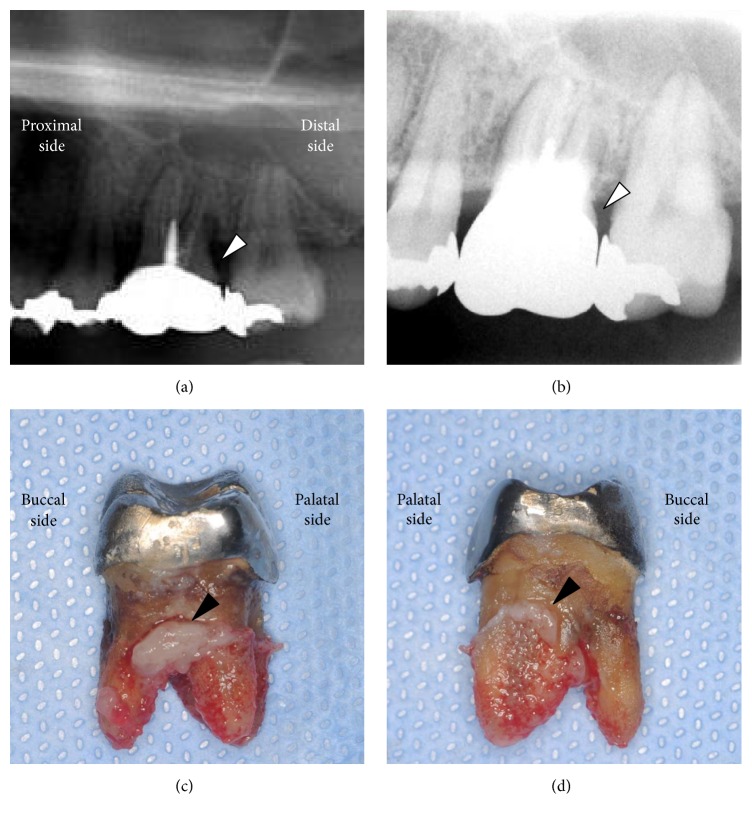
(a, b) Panoramic and dental radiographic images after embolization. The maxillary left first molar with crown and root filling material had a carious cavity on the distal side (white arrowhead). There was no defect in the alveolar bone. (c) The proximal and (d) distal sides of the extracted tooth. Cavities, remarkable calculus accumulation on the root surface, and infectious granuloma (arrowhead) were found.
